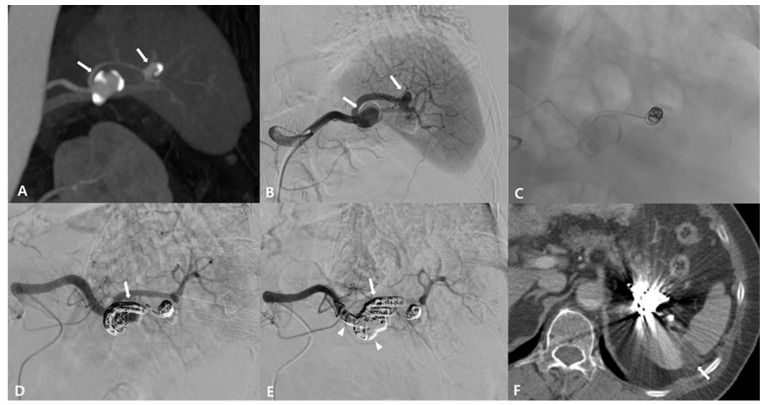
A 75-year-old woman with two splenic artery aneurysms. (A,B) Maximum-intensity-projection image of contrast-enhanced CT and selective splenic angiography show two SAAs (arrows). (C) A 6 mm 3D coil is placed in the smaller aneurysm in the superior polar branch. (D) A 5 mm 3D coil is placed in one outflow branch for initial framing (arrow). (E) Another 5 mm 3D coil (arrow) is placed in the other outflow branch, followed by additional 3D and non-3D coils placed in the aneurysm sac and inflow branch to achieve complete sac occlusion (arrowhead). (F) Follow-up CT at 2 months shows dense coil packing in both SAAs, with partial splenic infarction (arrow).
